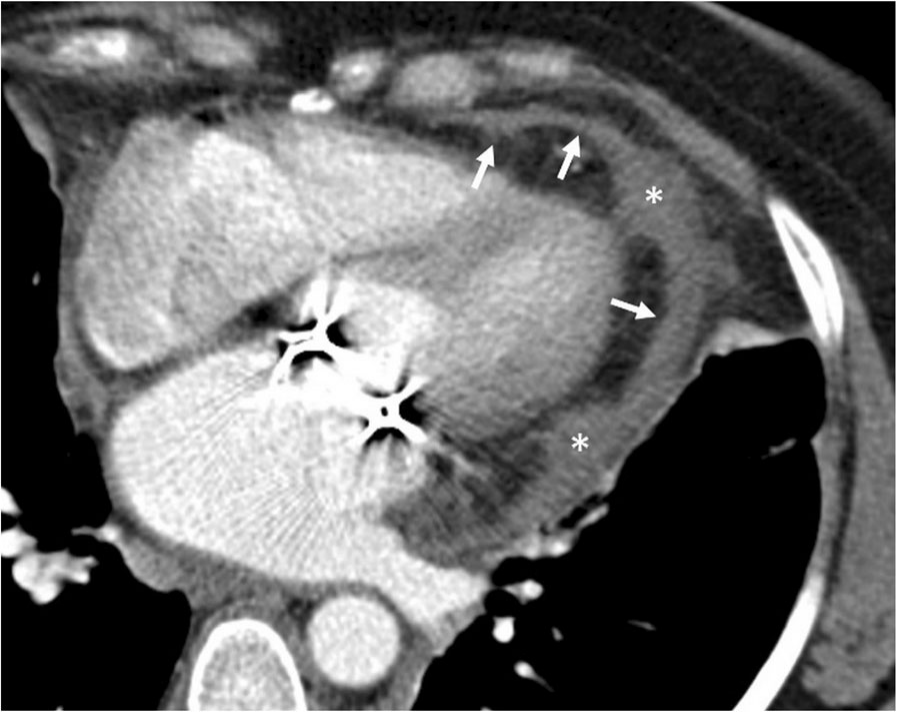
A 49-year-old woman with a previous history of mitral valve replacement surgery. Axial contrast-enhanced CT image shows a pericardial effusion (arrows) with irregular and nodular margins (asterisks). Post-surgical adhesions can mimic imaging signs encountered in pericardial metastasis
